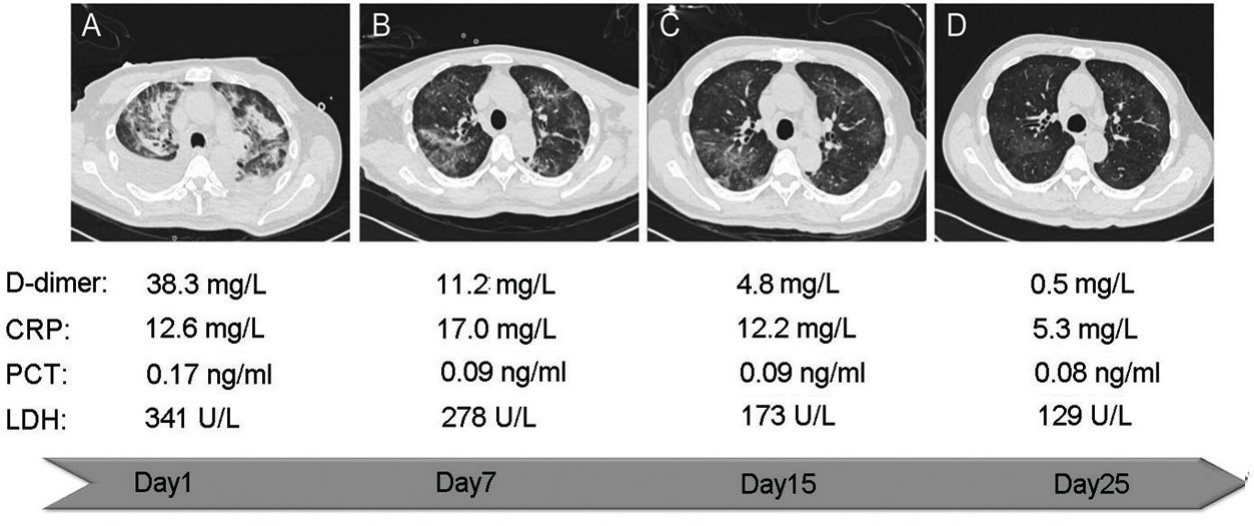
Representative computed tomography of a 62-year-old male patient during adjuvant antithrombotic treatment. Abidol and Ibuprofen was taken orally by the patient intermittently. After admission, the combination of anticoagulation was used (Aspirin enteric-coated tablets 100mg once a day and Clopidogrel bisulfate tablet 75mg once a day). The representative CT and the blood test results during the treatment were shown as A-D. (A) The infection was severe, and the diffuse distribution of the infection foci was dominated by bilateral pleural effusion. The D-dimer level was very high (38.3 mg/L). (B) The infection foci of the bilateral upper lung were reduced, and bilateral pleural effusion had been absorbed by itself. (C) The foci were reduced continuously and were mainly in the right upper lung at this time. (D) The infection foci were almost completely absorbed, while the D-dimer level of patient close to normal (0.5 mg/L).
